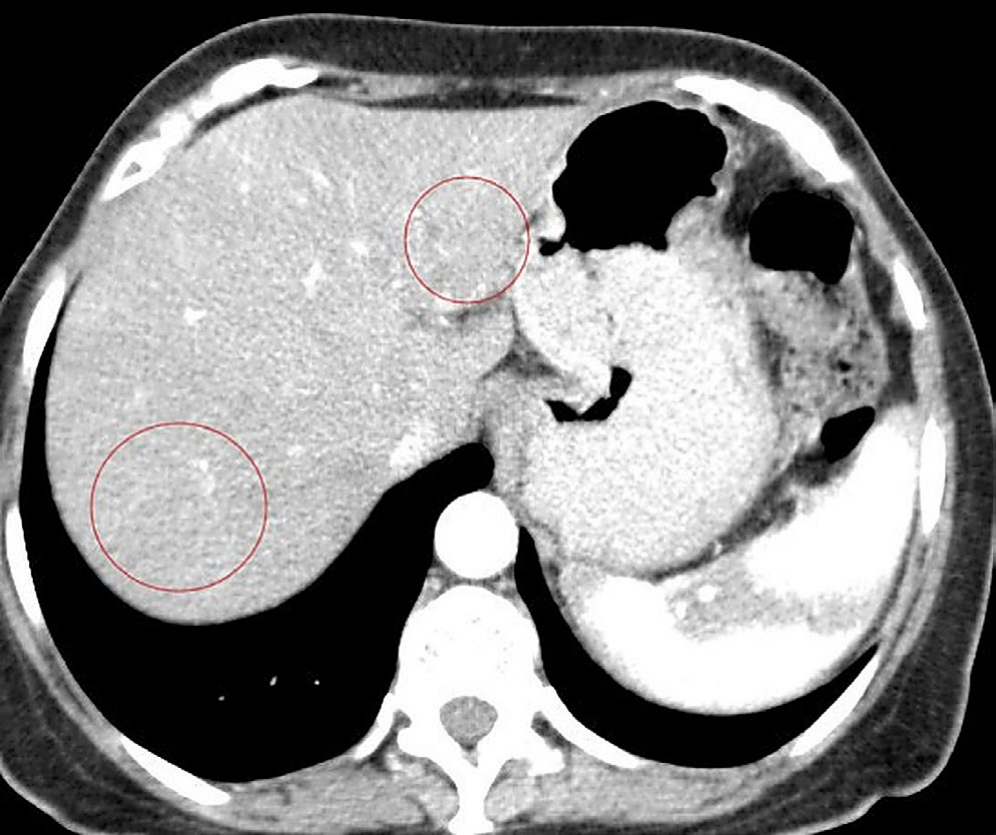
Follow‐up axial contrast‐enhanced computed tomography of the abdomen obtained 3 months following completion of the second treatment cycle demonstrates complete radiological response. No residual enhancing lesions are identified within either hepatic lobe (red circles denote the prior lesion locations).
